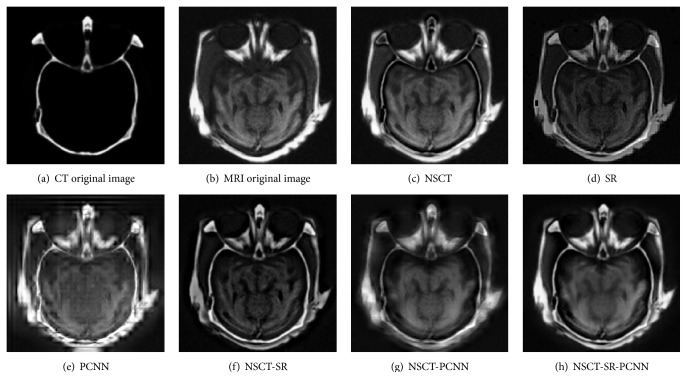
CT/MRI medical image fusion results.
